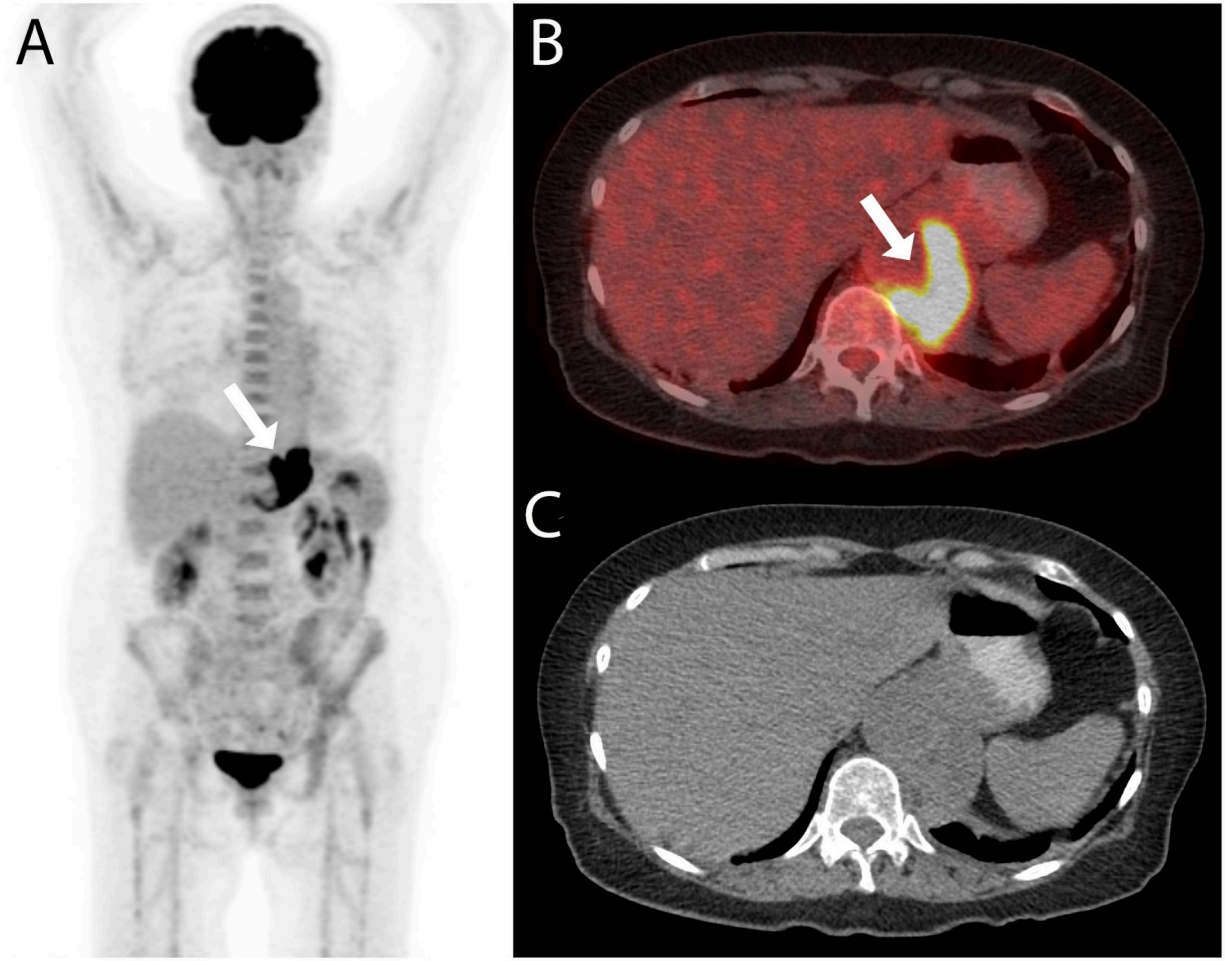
PET/CT with confirmed impact on management. A 71-year old woman was referred for PET/CT for staging of gastric cancer and with the question for an infectious aneurysm. The PET/CT examination (A: maximum intensity reconstruction, B: fused axial PET/CT image, and C: axial non-enhanced CT image) showed a FDG-avid mass adjacent to the abdominal aorta (white arrows in A and B). Both readers correctly rated the finding to be an infectious aneurysm; no gastric cancer was detected. The diagnosis was confirmed after biopsy (MAA caused by *Coxiella burnetii*) and the patient was treated with endovascular aortic repair and partial gastrectomy and lymphadenectomy. The patient was treated with antibiotics for 784 days and did not show any sign of recurrence at the last clinical visit 293 days after the end of antimicrobial therapy.
